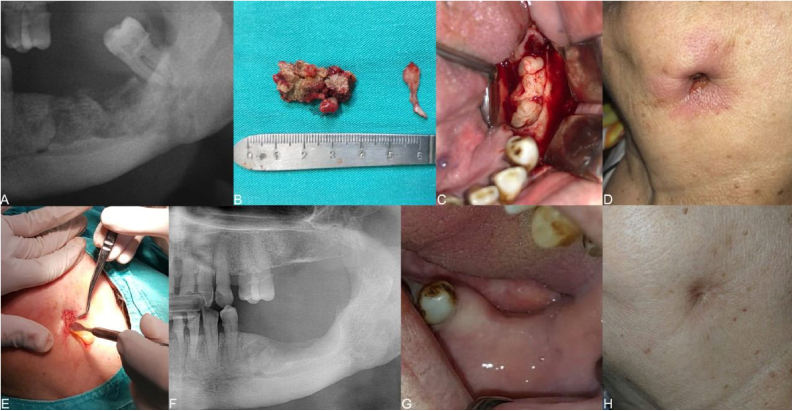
The clinical presentation of medication‐related osteonecrosis of the jaw lesion in a 79-year-old female patient with metastatic breast cancer (stage 3): (A) initial panoramic radiograph of the lesion extending to the mandibular basis in the left posterior mandible; (B) necrotic bone was completely removed; (C) placement of the leukocyte- and platelet-rich fibrin membrane in the surgical area; (D) initial clinical photograph showing a skin sinus tract at the submandibular area; (E) placement of the leukocyte- and platelet-rich fibrin membrane in the skin sinus tract; (F) follow-up at 12-months, postoperative panoramic radiograph of the patient after surgery of the mandibular left molar region; (G) follow-up 3-months, postoperative clinical photograph revealing healing of surgical area; (H) follow-up 3-months, clinical photograph demonstrating healing of the skin sinus tract.
